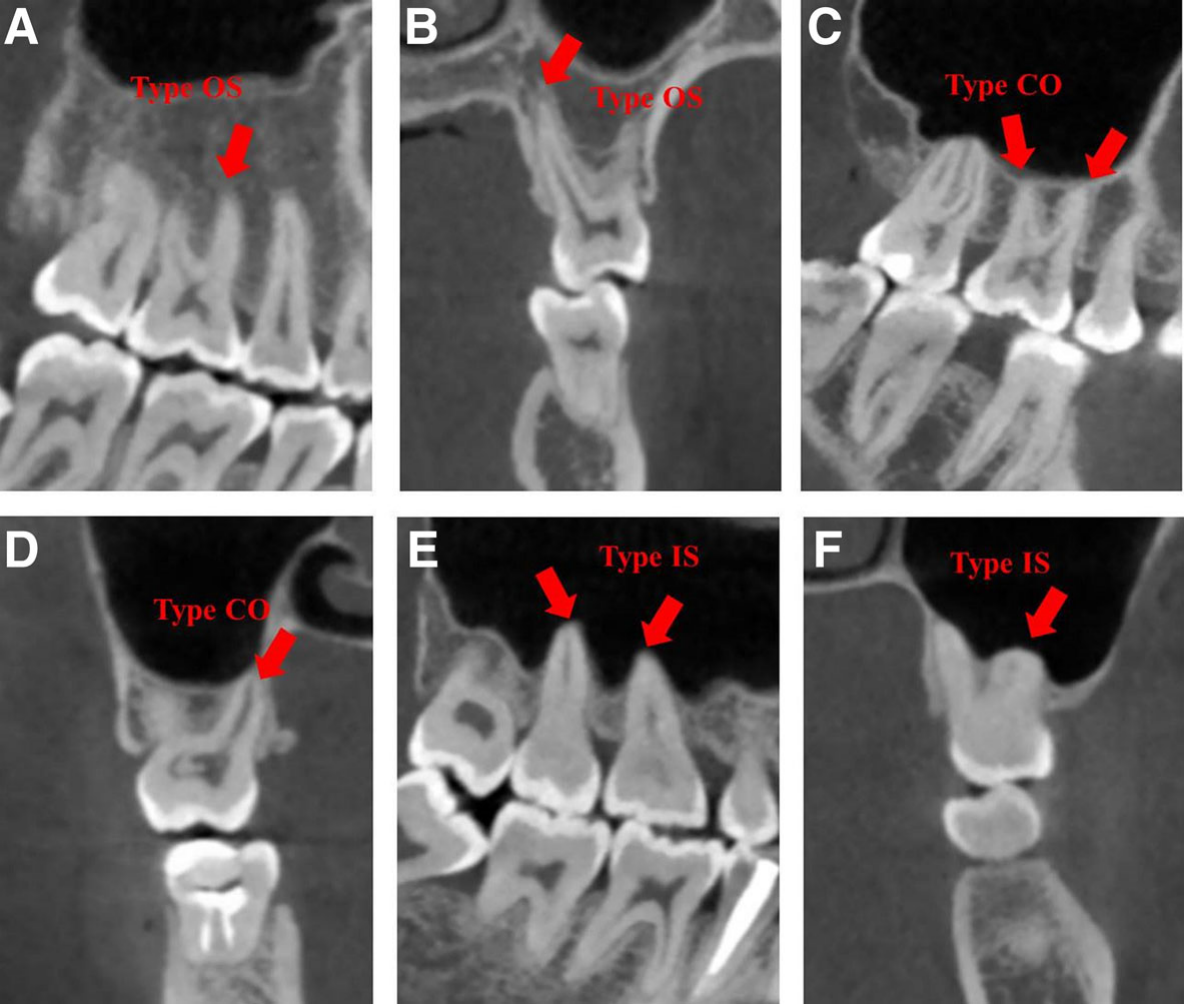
CBCT images of three vertical relationships between maxillary posterior teeth and MSFs in sagittal (a, c and e) and coronal planes (b, d and f). a Type OS: MBR of a right MFM; b Type OS: PR of a left MFM; c Type CO: MBR and DBR of a right MFM; d Type CO: PR of a right MSM; e Type IS: PRs of right maxillary molars; f Type IS: MBR of a left MSM
